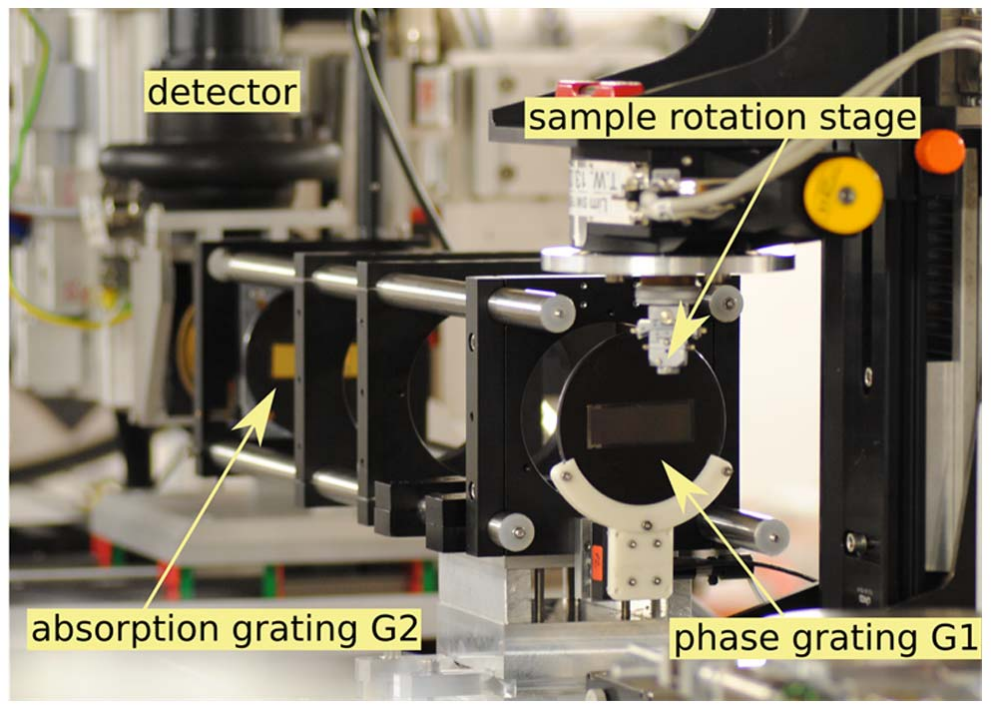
Photograph of the Interferometer Setup at Beamline ID19, ESRF, Grenoble, France. Monochromatic, coherent X-rays travel through the sample and introduce distortions in the interference pattern created by the phase grating. Those are evaluated by a lateral movement of the phase grating located behind the sample. Photograph adapted from I. Zanette, *Interférometrie X à réseaux pour l'imagerie et l'analyse de front d'ondes au synchrotron*, PhD thesis, University of Grenoble, 2011.
